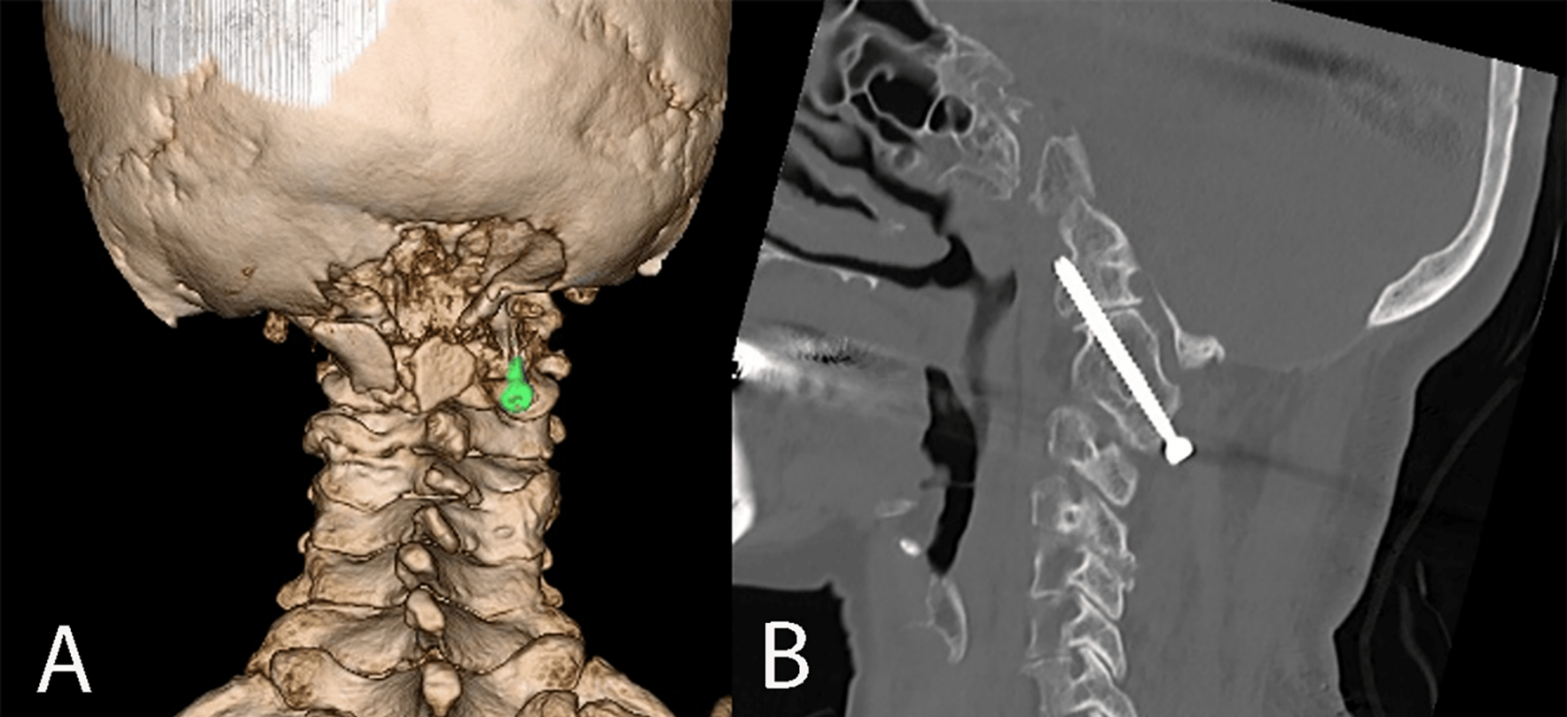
Case 1. Occipitocervical fusion with a hemilateral TAS and iliac bone grafting. (A) Three-dimensional CT; (B) CT sagittal section. TAS: transarticular screw; CT: computed tomography.
